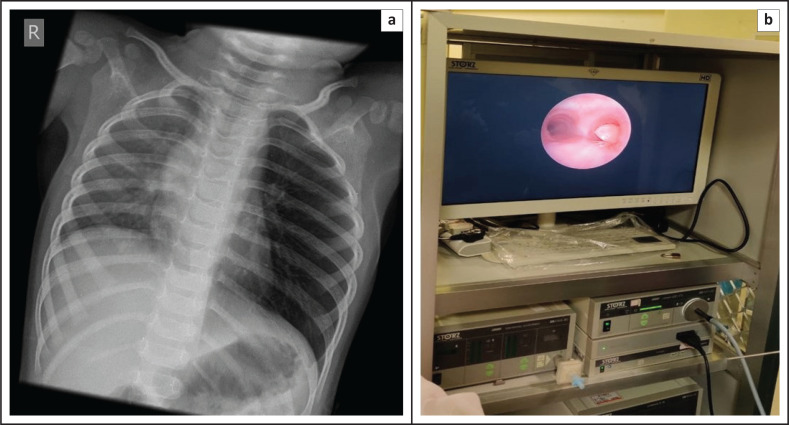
Chest radiograph (a) of a 2-year-old child who aspirated a peanut into the left main bronchus with resultant air trapping and hyperinflation of the left lung. Bronchoscopy (b) confirmed the aspirated peanut in the left main bronchus.
